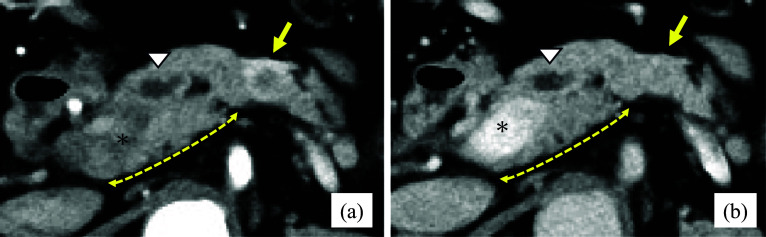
Preoperative contrast-enhanced CT; Case 2. a Arterial phase: CP and a tumor with contrast effect in the pancreatic tail (yellow arrow). b Portal phase: suprasplenic CP with anteportal MPD. The pancreatic duct did not run through the pancreatic parenchyma on the dorsal side of the PV. Dotted yellow line: range of the dorsal parenchyma. The asterisk: the PV. White triangle: MPD. Yellow arrow: Tumor with a contrast effect
